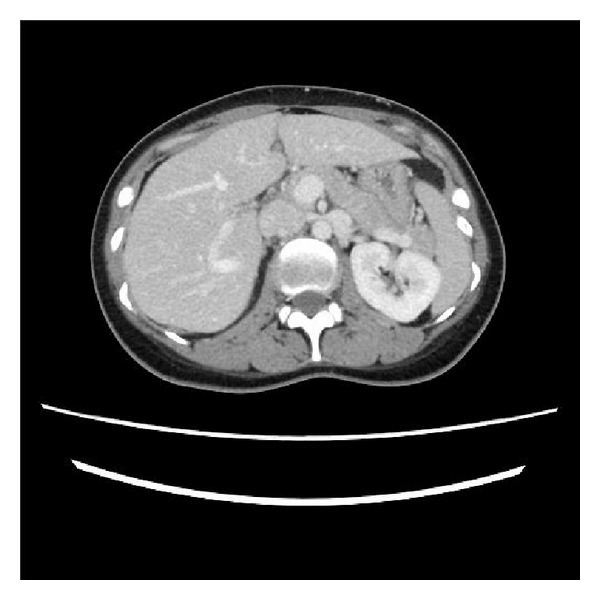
Enhanced computed tomography of transverse section. Left renal vein becomes compressed between the superior mesenteric artery and the aorta.
